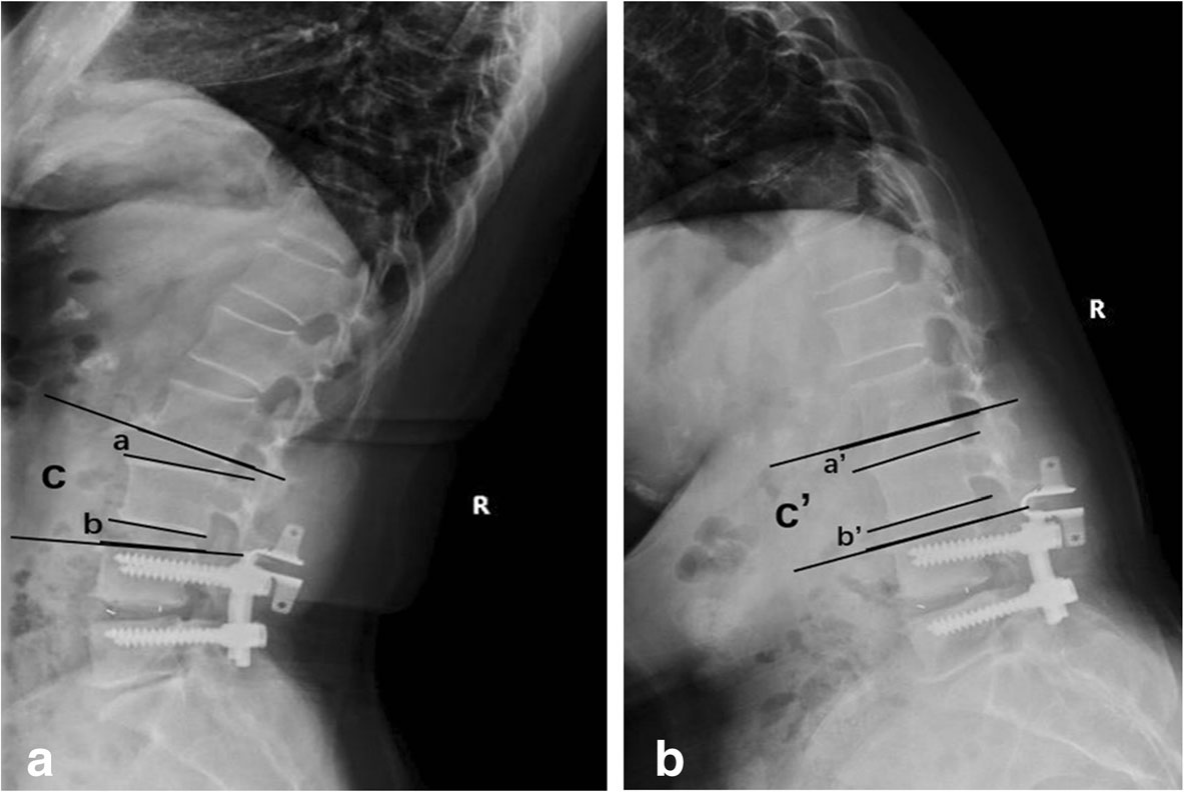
Lateral flexion and extension lumbar X-ray showing radiological indices studied. Adjacent intervertebral mobility (L2–L3) = extension angle (a)–flexion angle (a’). Intervertebral mobility (L3–4) with Coflex insertion = extension angle (b)–flexion angle (b’). General adjacent segment mobility (GASM) at L2–4 = extension angle (c)–flexion angle (c’)
